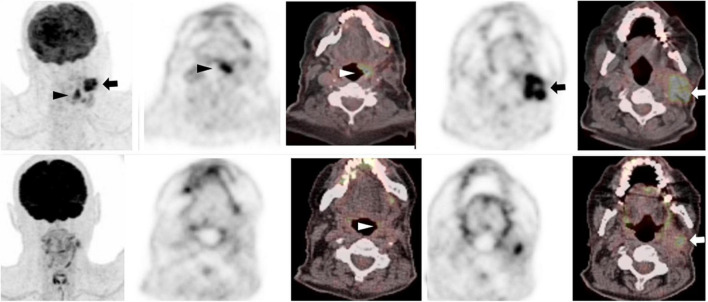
75-year-old man with left tongue base squamous cell carcinoma. Baseline ^18^F-FDG PET/CT (top row – zoomed MIP image left, axial PET and fused PET/CT image at level of primary tumor (middle columns) and neck level II lymphadenopathy (right columns) show an intensely metabolically active left tongue base tumor (arrowhead) with metabolically active metastatic left neck lymphadenopathy (arrow). Follow-up PET following radical chemoradiotherapy (corresponding images, bottom row) showed complete metabolic response at the primary tumor (white arrowhead, bottom row), with a persistent metabolically active left neck lymph node, Hopkins score 5, consistent with residual tumor (white arrow, bottom row).
